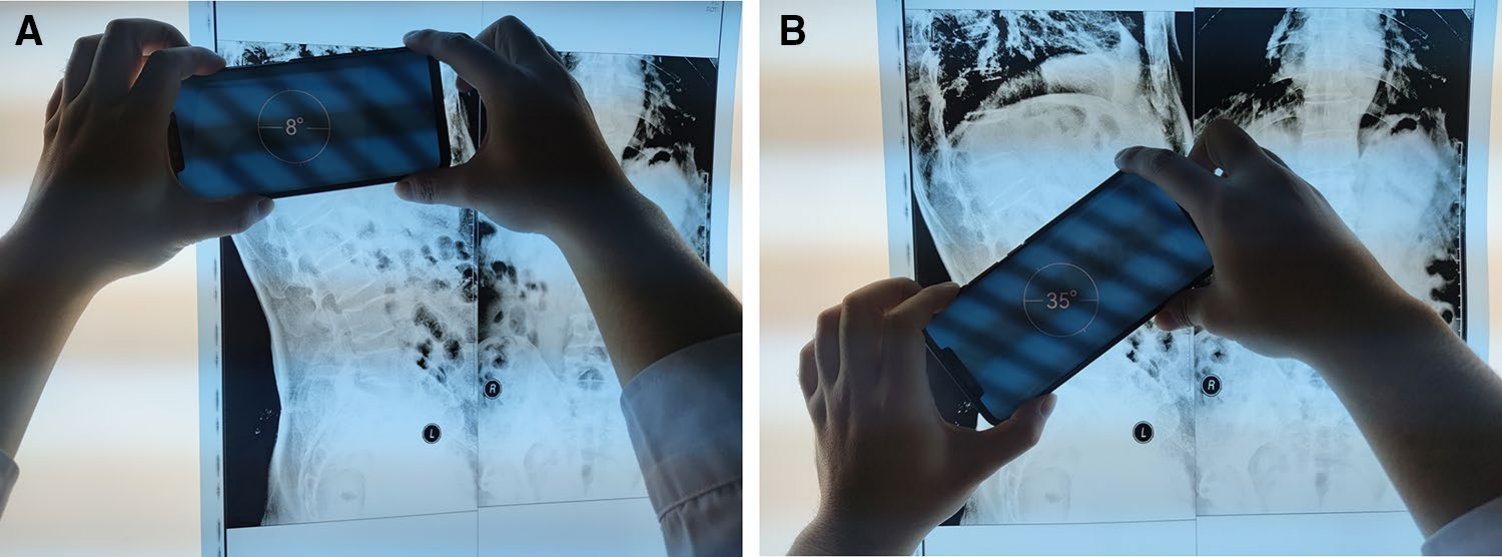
The integrated inclinometer in a smartphone was used to measure thoracolumbar kyphosis on lateral plain X-ray films. (A) First, the vertebral body of T10 was confirmed, a certain edge of the smartphone was aligned to the upper endplate of T10, and the angle shown on the smartphone screen was recorded. (B) Second, the vertebral body of L2 was confirmed, the same edge of the smartphone was aligned to the lower endplate of L2, and the angle shown on the smartphone screen was recorded.
